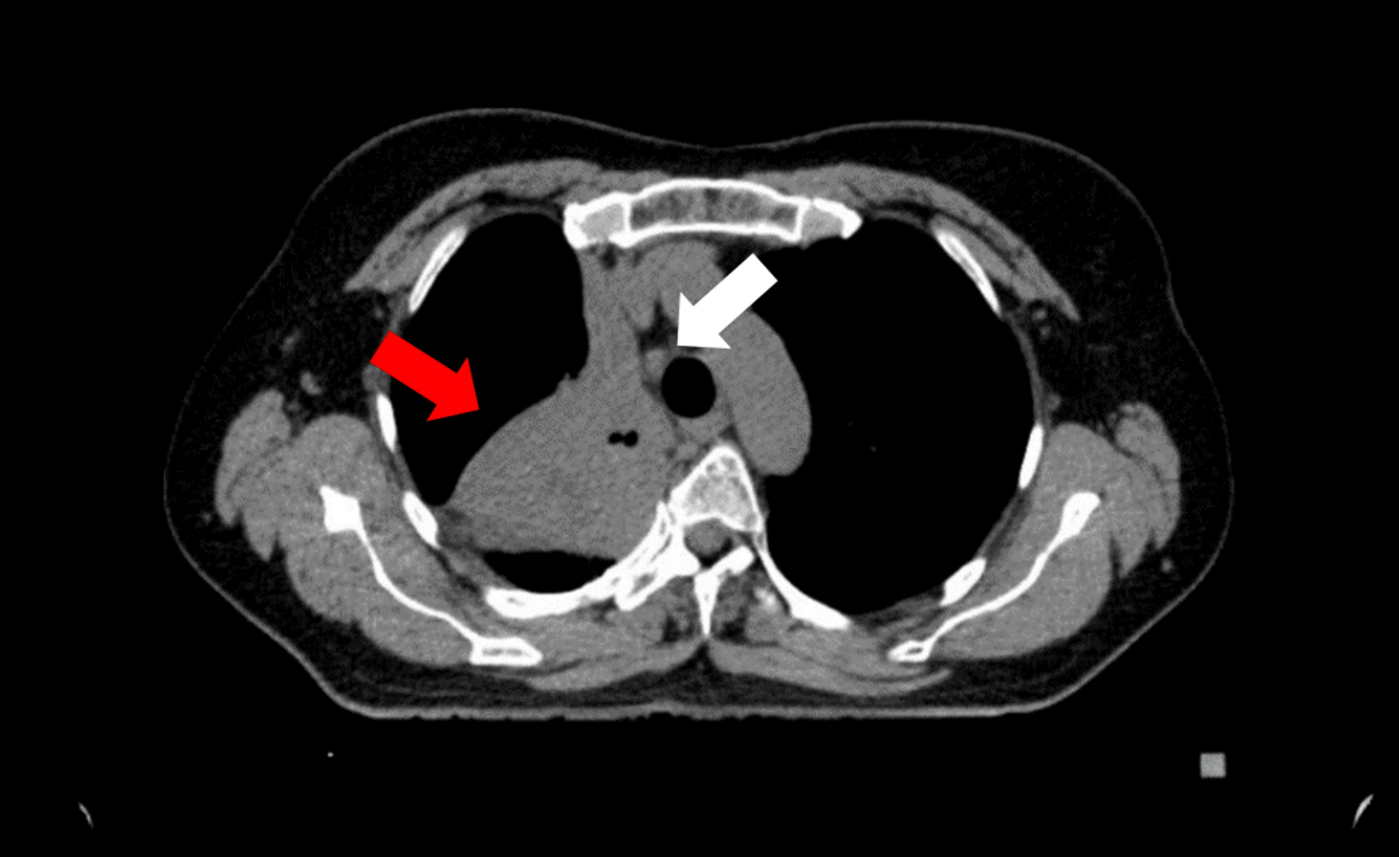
Chest CT image showing the tumor (indicated by the red arrow) and the paratracheal lymph node (indicated by the white arrow)
